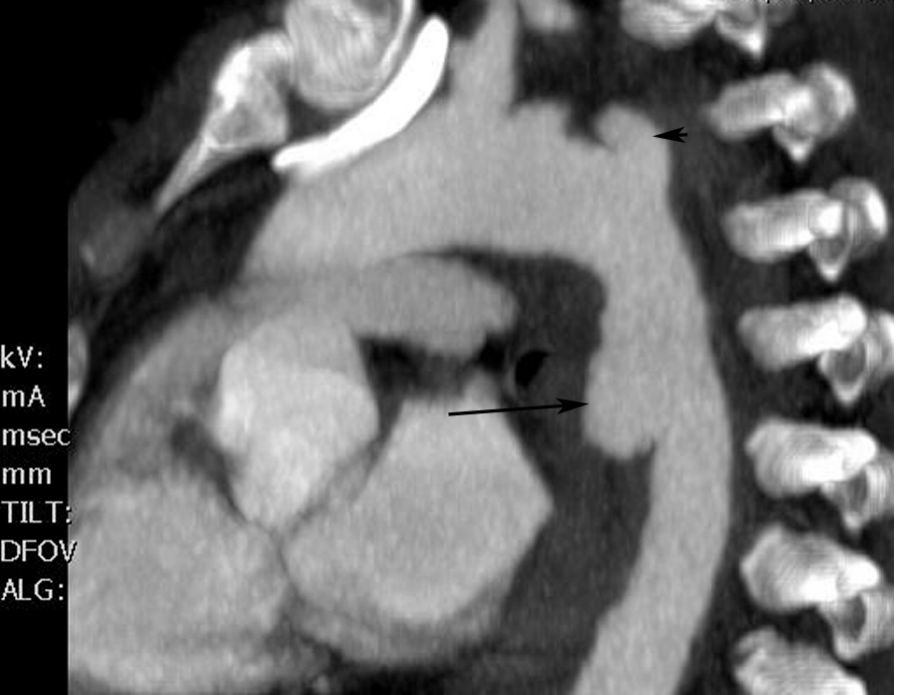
Reconstructed computed tomography with contrast depicting aortic injury with pseudoaneurysm (arrow). Arrowhead indicates proximal left subclavian artery.
